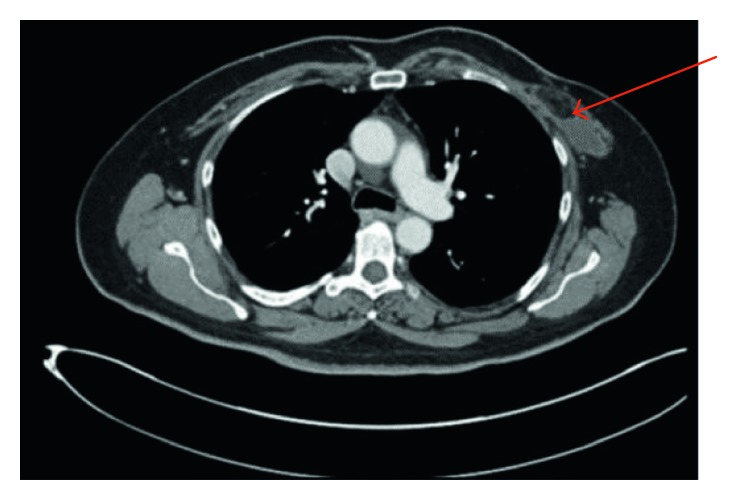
CT scan of chest: large fluid collection in the left chest wall measuring 12.9 × 2.8 × 10.1 cm.
